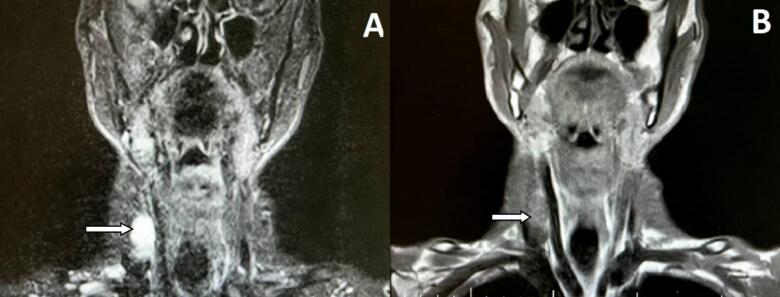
Magnetic Resonance Imaging of the neck, A) a hyperintense and hetrogenous mass on T2-weighted B) a regular Isointense mass on T1-weighted.
